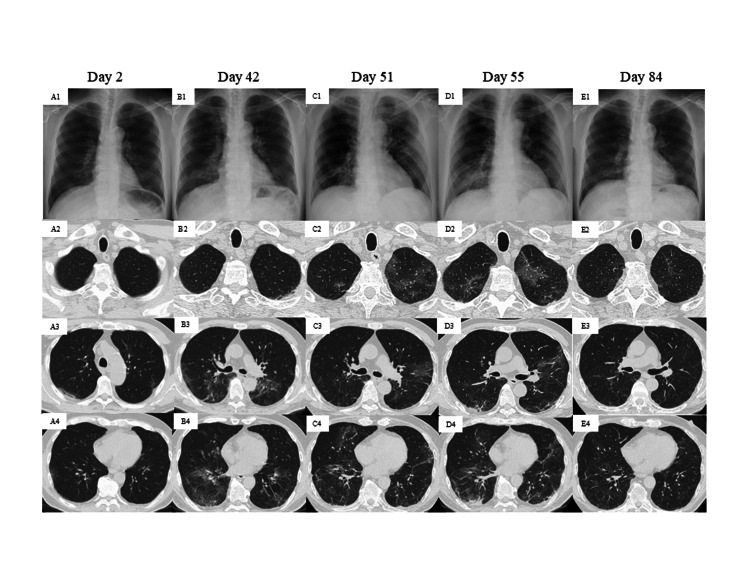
Radiographic progression of the disease on chest X-ray and CT Chest X-ray and CT findings revealed subtle subpleural GGOs in both upper lung fields on day 2 after the onset of coronavirus disease 2019 (A3). By day 42, bilateral GGO appeared in both lower lung fields (B1-B4). On day 51, GGO was observed in the left upper (C2) and lingula lung fields (C3), accompanied by a reduction of GGO in both lower lung fields (C4). On day 55, subpleural consolidation and relapse of GGO in the right lower lung fields were noted (D1-D4). By day 97, these pulmonary opacities had nearly disappeared (E1-E4). GGO, ground-glass opacity
